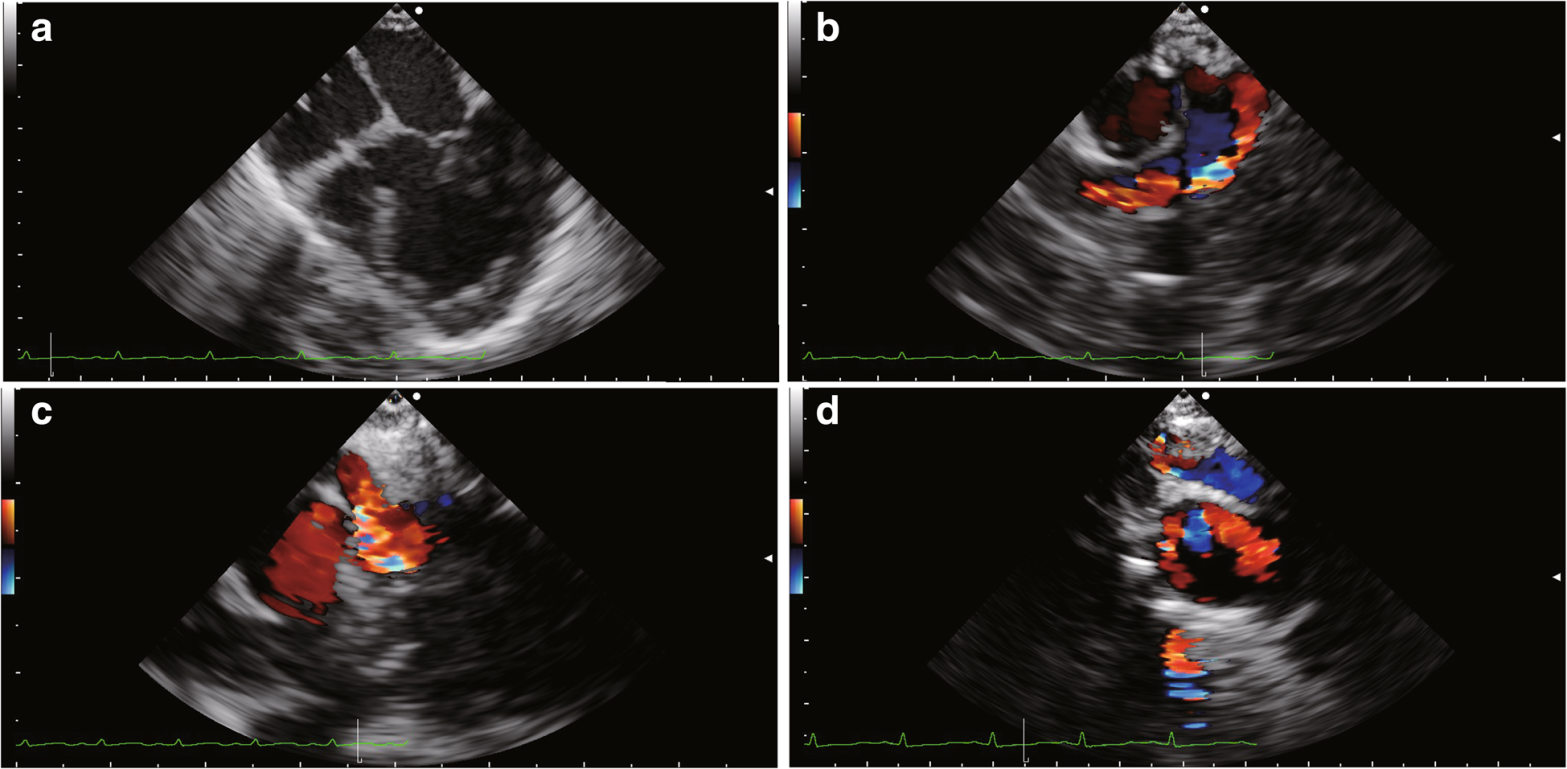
Intraoperative transesophageal echocardiographic images. a Midesophageal four-chamber view demonstrating tricuspid atresia, a hypoplastic right ventricle, and VSD. b Midesophageal right ventricle inflow–outflow view using color Doppler imaging demonstrating pulmonary stenosis that caused a dissipative flow in the main pulmonary artery before BTS. c Midesophageal ascending aortic short-axis view using color Doppler imaging demonstrating an antegrade flow from the completely severed main pulmonary artery to both the right and left pulmonary arteries due to BTS, which connected the brachiocepharic artery to the main pulmonary artery. d, Midesophageal ascending aortic short-axis view demonstrating the retrograde flow from the right pulmonary artery to the left pulmonary artery due to the BCPS, which connected the superior vena cava to the right pulmonary artery
